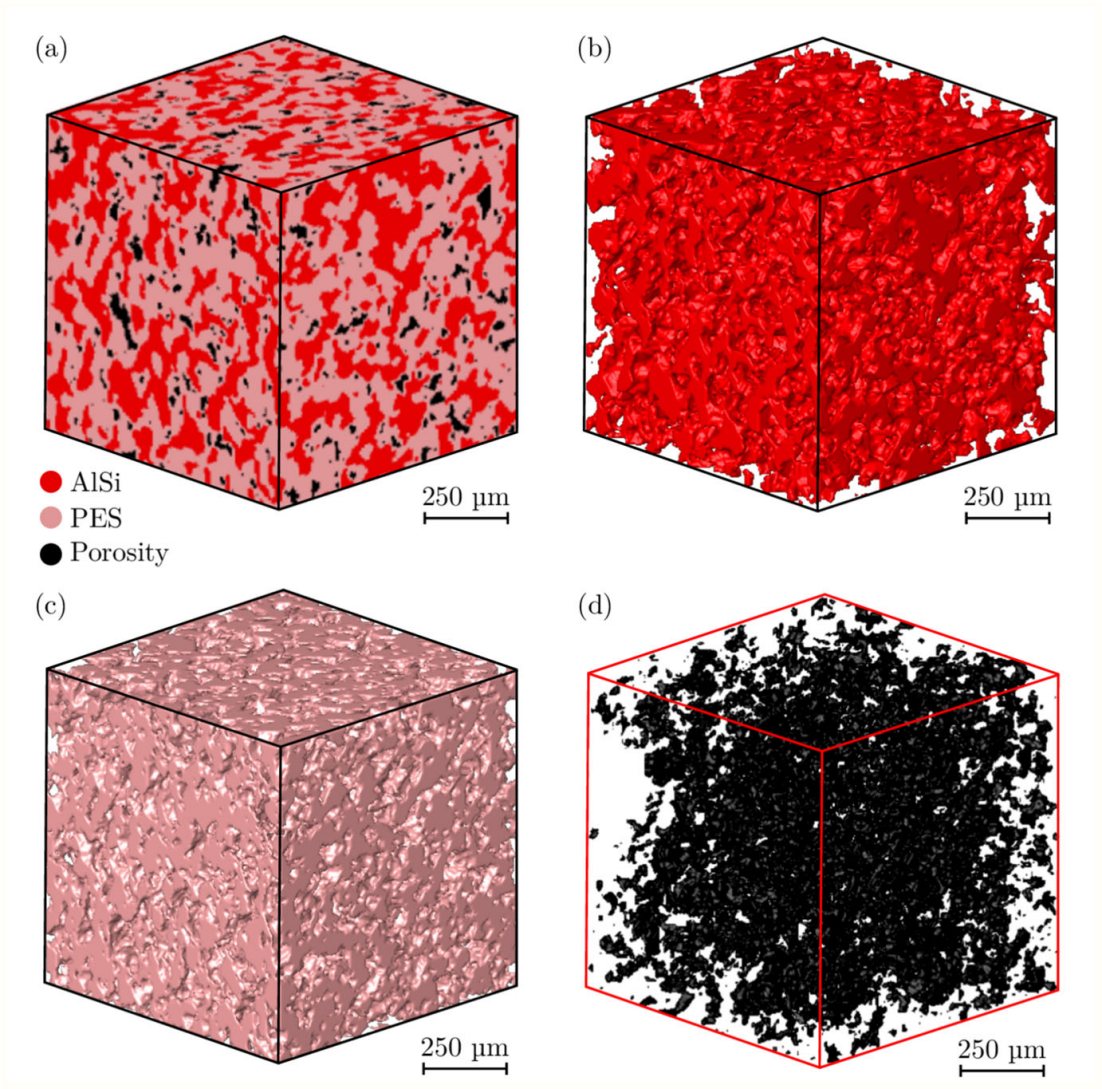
RVE extracted from X-ray CT scan of an as-sprayed sample, with 1 mm edge length. (a) RVE, (b) AlSi phase, (c) PES phase, (d) Porosities
